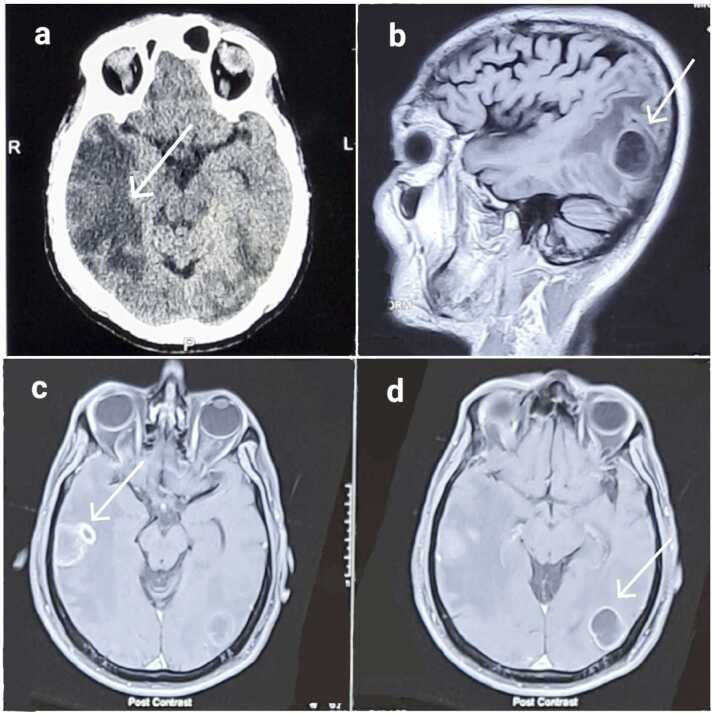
Neuroimaging of tuberculomas of Case 3 (a) Axial non-contrast CT brain showing a hypodense lesion in the right parietal region (arrow) and in left parieto-occipital region. (b) Sagittal T1-weighted MRI (non-contrast) demonstrating a right parietal lesion suggestive of tuberculoma (arrow). (c) Axial post-contrast T1-weighted MRI showing ring-enhancing right parietal tuberculoma with surrounding edema (arrow). (d) Axial post-contrast T1-weighted MRI showing ring-enhancing left parietal tuberculoma (arrow).
